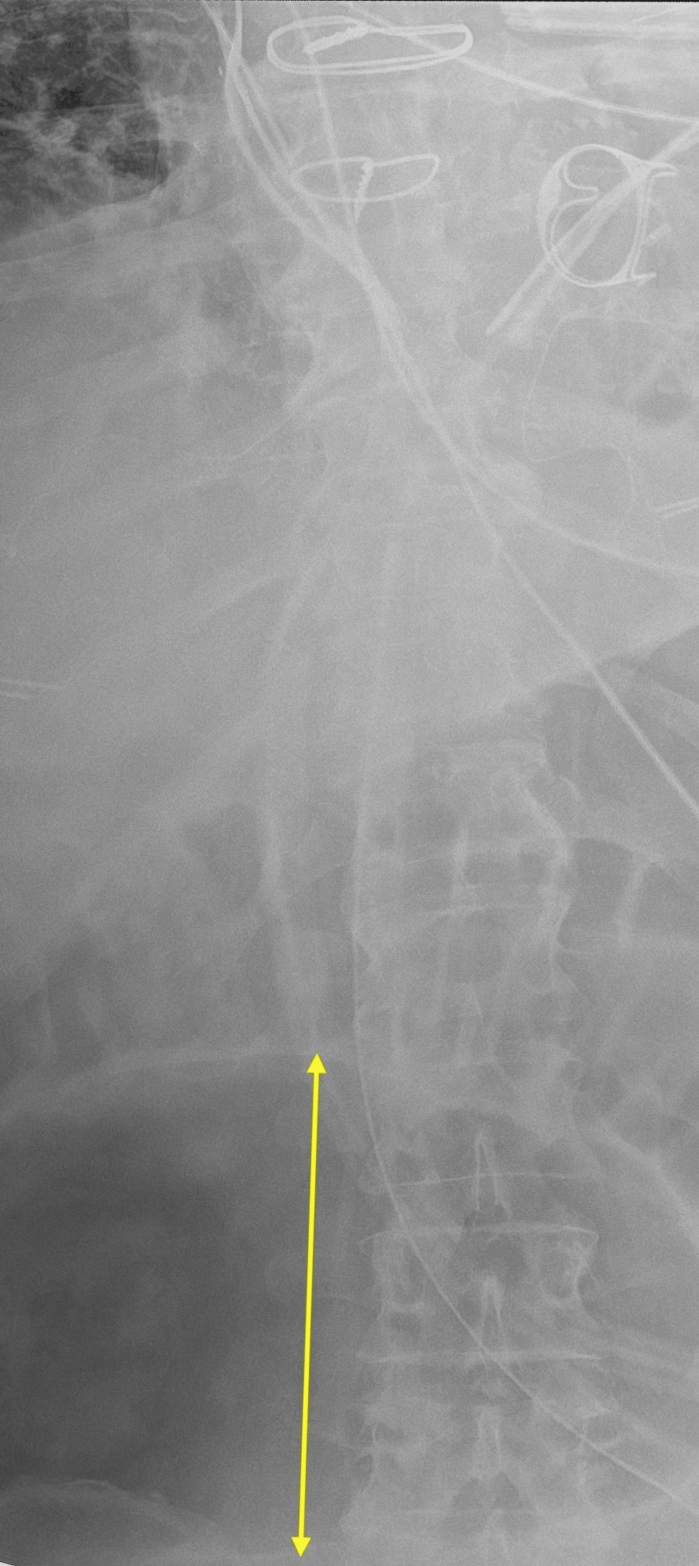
Abdominal radiograph An enlarged cecum is shown, measuring 12 cm (arrow), concerning for cecal bascule.
